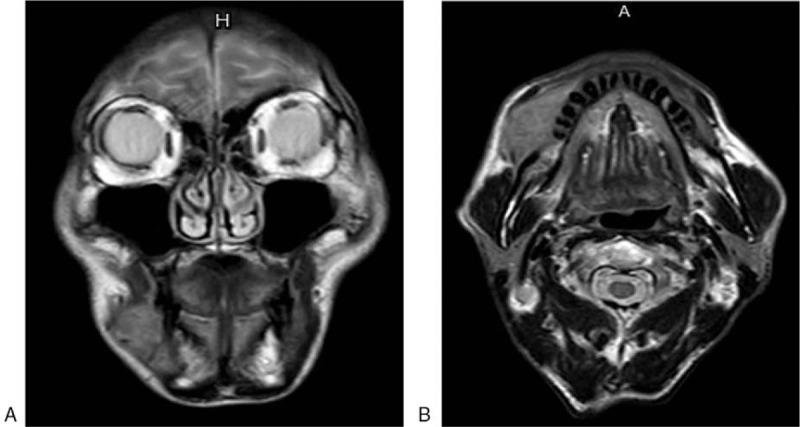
Magnetic resonance images of the neck presented a mass shadow on the right cheek with no clear boundary and a size of approximately 4.0 × 2.2 cm. (A. *axial view*; B. *coronal view*).
